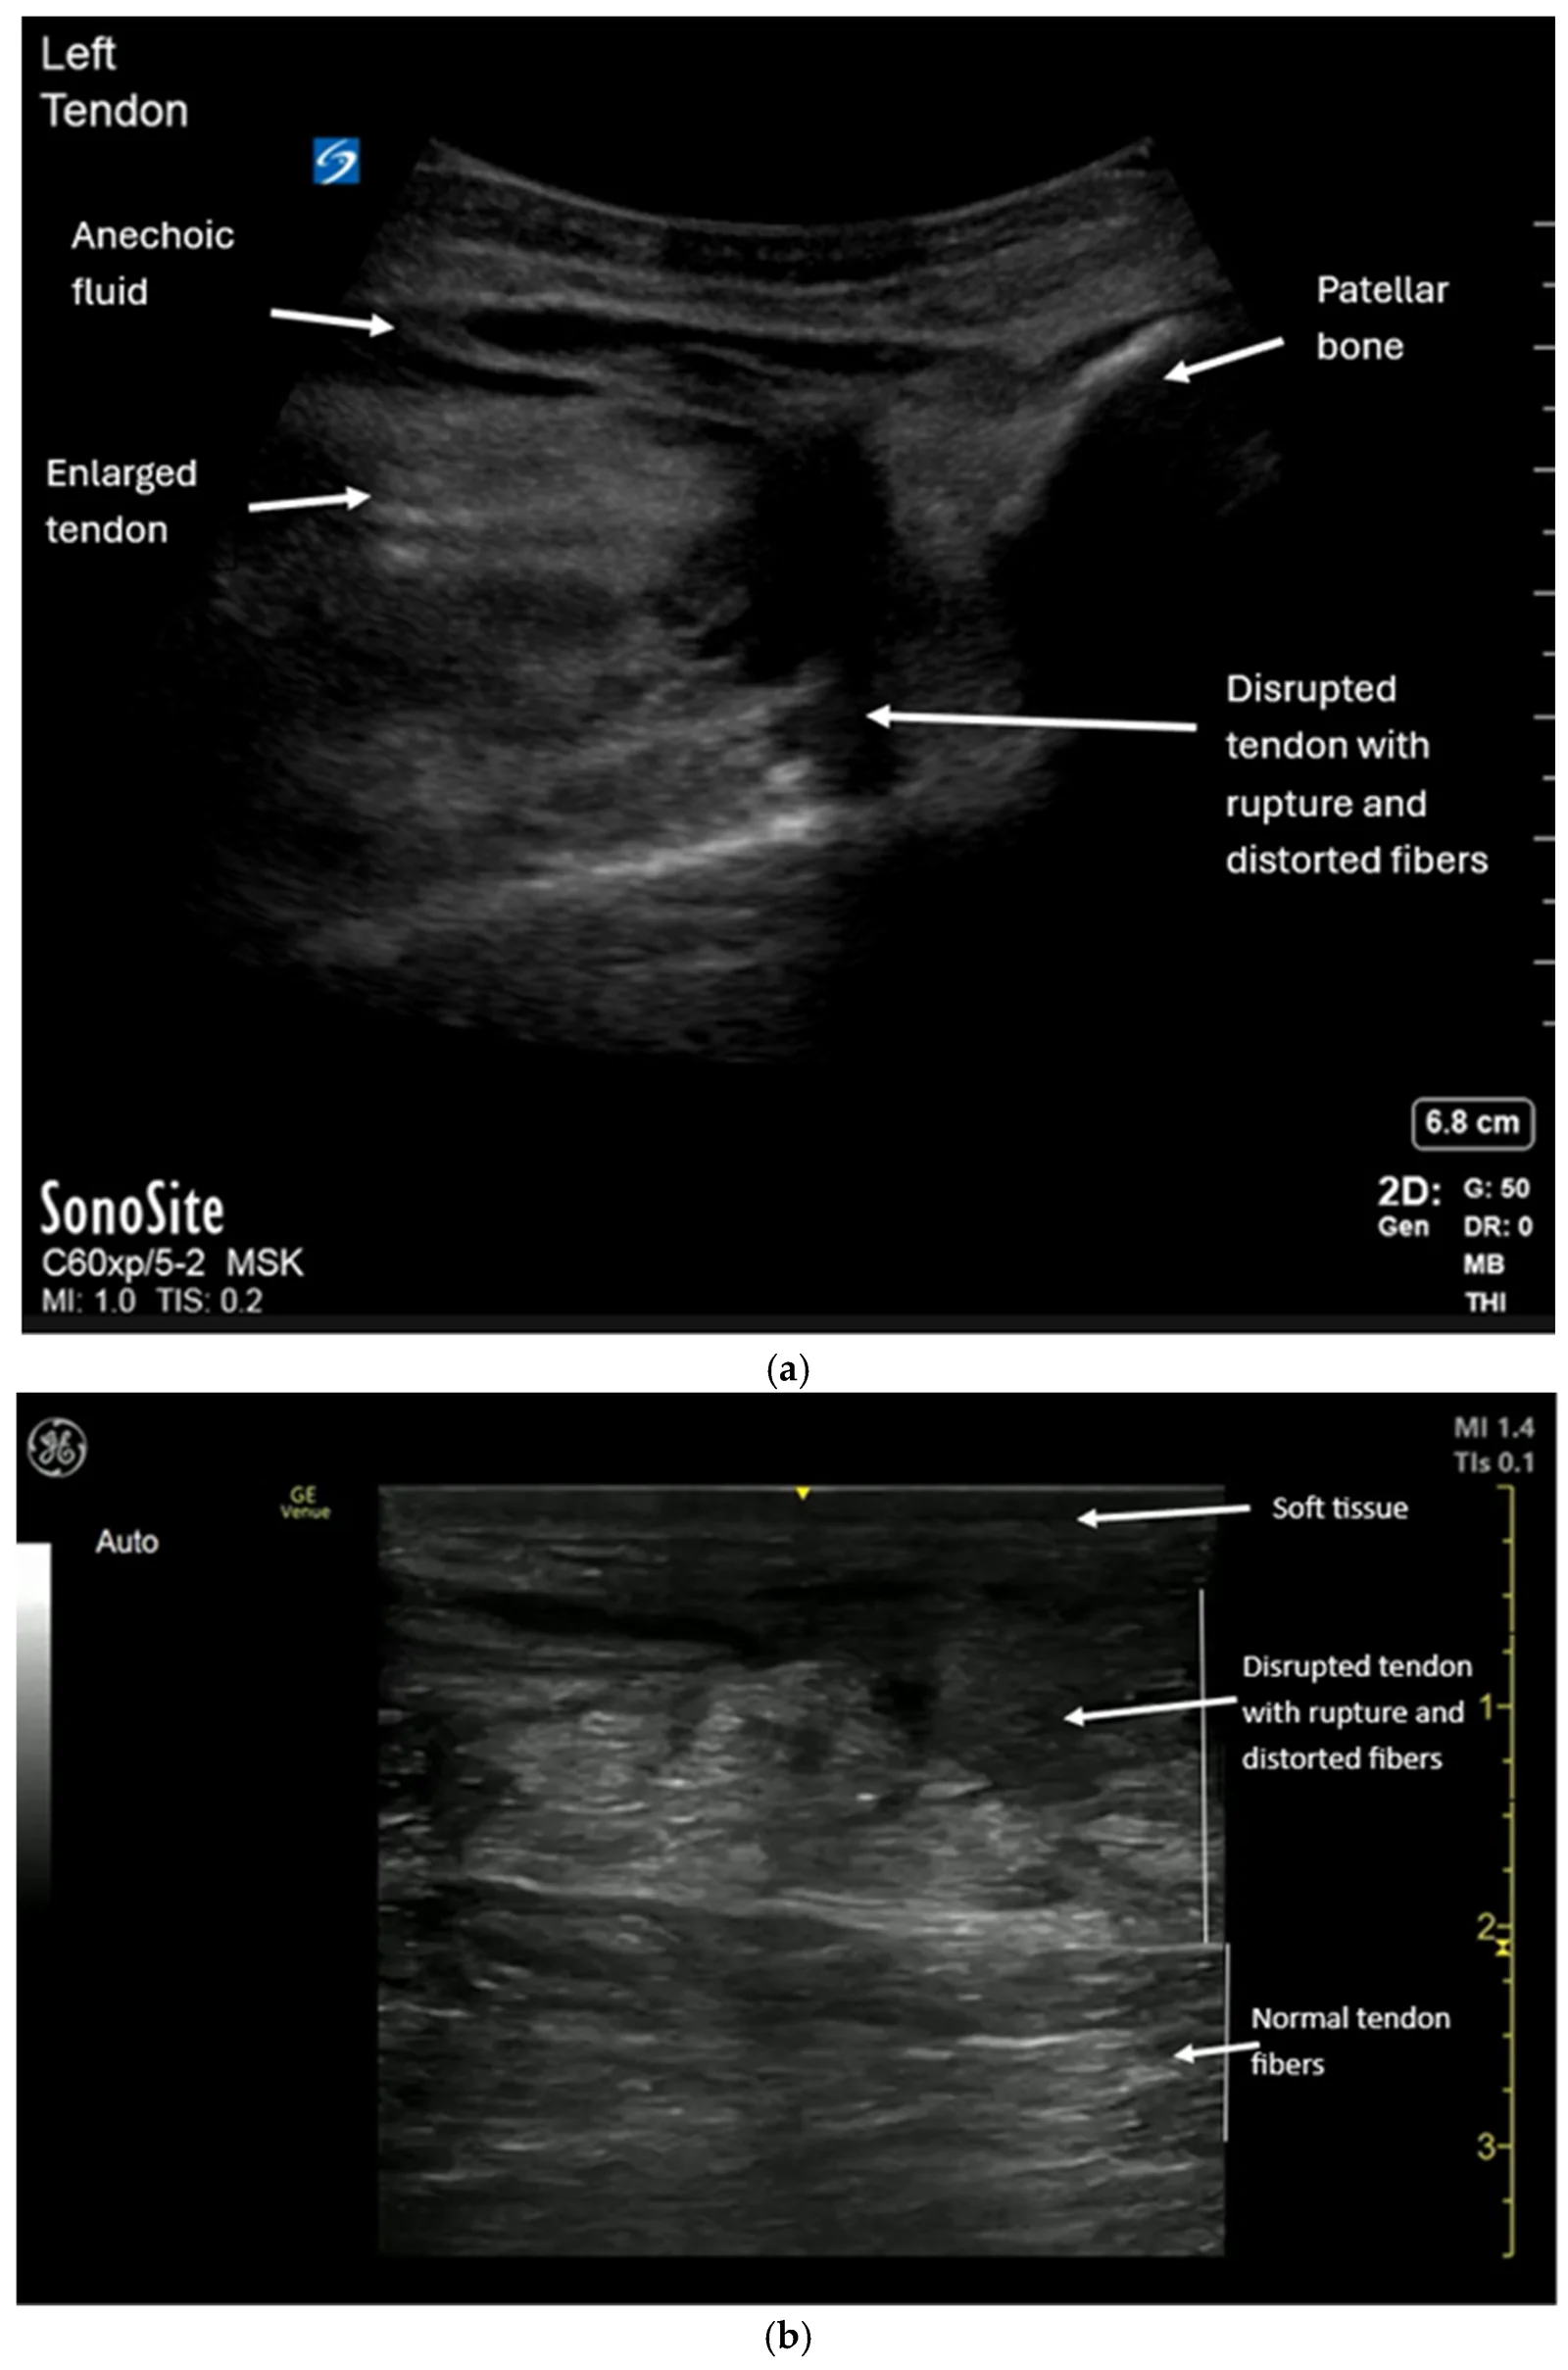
Dynamic point-of-care ultrasound (POCUS) can be performed at the patient’s bedside, provides rapid diagnosis, and helps guide clinical management for musculoskeletal injuries, especially in emergency department (ED) patients with recent trauma. (a) demonstrates a complete quadriceps tendon rupture with an enlarged tendon and tendon retraction away from the patellar bone, which cannot be easily seen on radiography or computed tomography [1]. Background: Quadriceps tendon rupture is a lower limb injury that involves suprapatellar pain, loss of knee extension, and a difficult rehabilitation course [1,2,3]. Tendon rupture occurs during eccentric contraction of the quadriceps with knee flexion against an active quadriceps muscle or when a person falls backwards with a planted foot [3,4]. Incidence is 1.37 of 100,000 cases and more frequent in patients over 40 years old [3,4]. Tendon rupture can be spontaneous, particularly in patients with predisposing risk factors such as prior tendon injury, underlying tendinopathy, or comorbidities such as rheumatologic disease, chronic kidney disease, or diabetes mellitus [4,5]. Clinical diagnosis of complete rupture includes distal thigh tenderness, a suprapatellar hematoma, and inability to actively extend the knee. Clinical presentation: These example images are from a healthy 34-year-old male who presented to the ED with acute left knee pain and swelling after landing on his left knee while playing basketball three days prior (a). He felt a pop with immediate pain and subsequent swelling. On examination, he had severe tenderness at his left distal thigh/proximal knee with a deformity superior to his patella. His pain was significantly worse with knee movement, and he was unable to stand and bear weight on the leg. Lower extremity strength, sensation, and distal pulses were normal, but he could not extend his left knee. Diagnostic imaging findings: An X-ray of his left knee showed a joint effusion with soft tissue swelling anterior to the patella but no acute fracture. As shown in (a), POCUS demonstrated a disrupted left distal quadriceps tendon with anechoic fluid, tendon enlargement near the insertion site, and tendon fiber distortion consistent with a complete tendon rupture (Supplementary Video S1). This is distinct from partial tears, which would show only partial disruption of the tendon, with hyperechoic fibers still attached to the bone distally in the unaffected portion of the tendon (b) (Supplementary Video S2). Diagnostic imaging is paramount to confirming tendon rupture diagnosis, and imaging availability, operator expertise, and clinical pathways vary across institutions. Imaging is important for injury confirmation and severity grading to guide management [3]. Radiography may show an inferiorly displaced patella with a patellar length ratio < 0.8, but this finding is not sensitive or specific [4]. Magnetic resonance imaging (MRI) is the gold standard but may be difficult to obtain in emergent or primary clinic settings, whereas performing POCUS can be a facile tool to evaluate for tendon rupture at the patient’s bedside for immediate critical management decisions [3,4,6].
